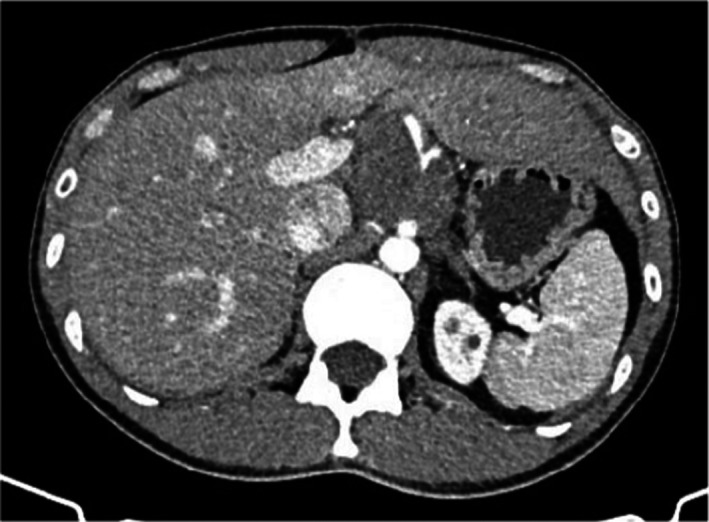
Patient 1: a recurrent tumor diagnosed 3 months after surgery surrounded the superior mesenteric artery and abdominal aorta. Enhanced CT scan showed a round solid‐cystic soft‐tissue mass with irregular enhancement surrounding the coeliac trunk and superior mesenteric artery.
